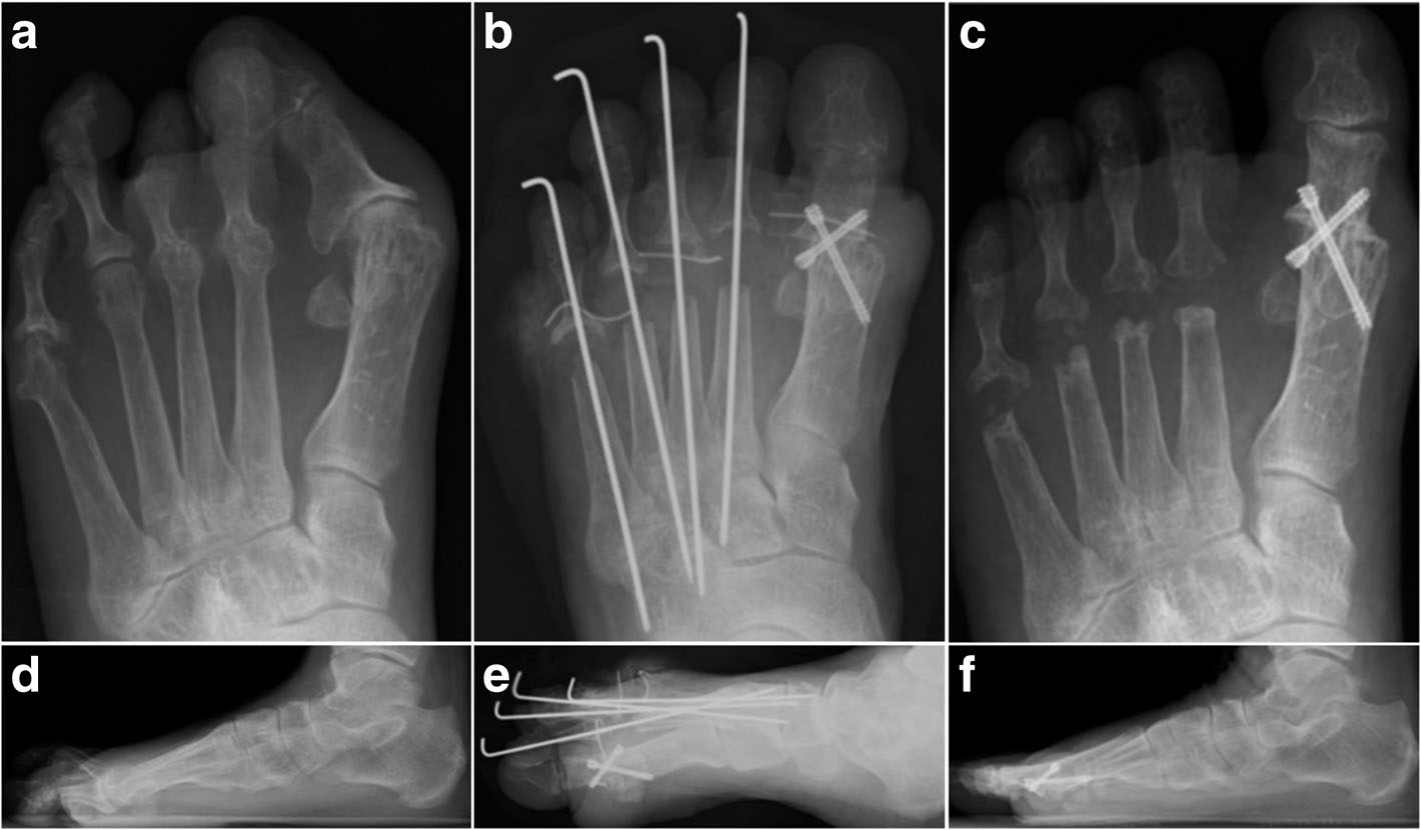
Representative radiographs. Preoperative AP (a) and lateral (d) radiographs showing typical forefoot deformities including hallux valgus, claw toe and MTP subluxation of second to fourth toes, and the quintus varus. Postoperative AP (b) and lateral (e) radiographs showing acceptable alignment of first MTP joint and no MTP subluxation of lessor toes. Radiographs five years after operation still indicate acceptable alignment (c, f)
